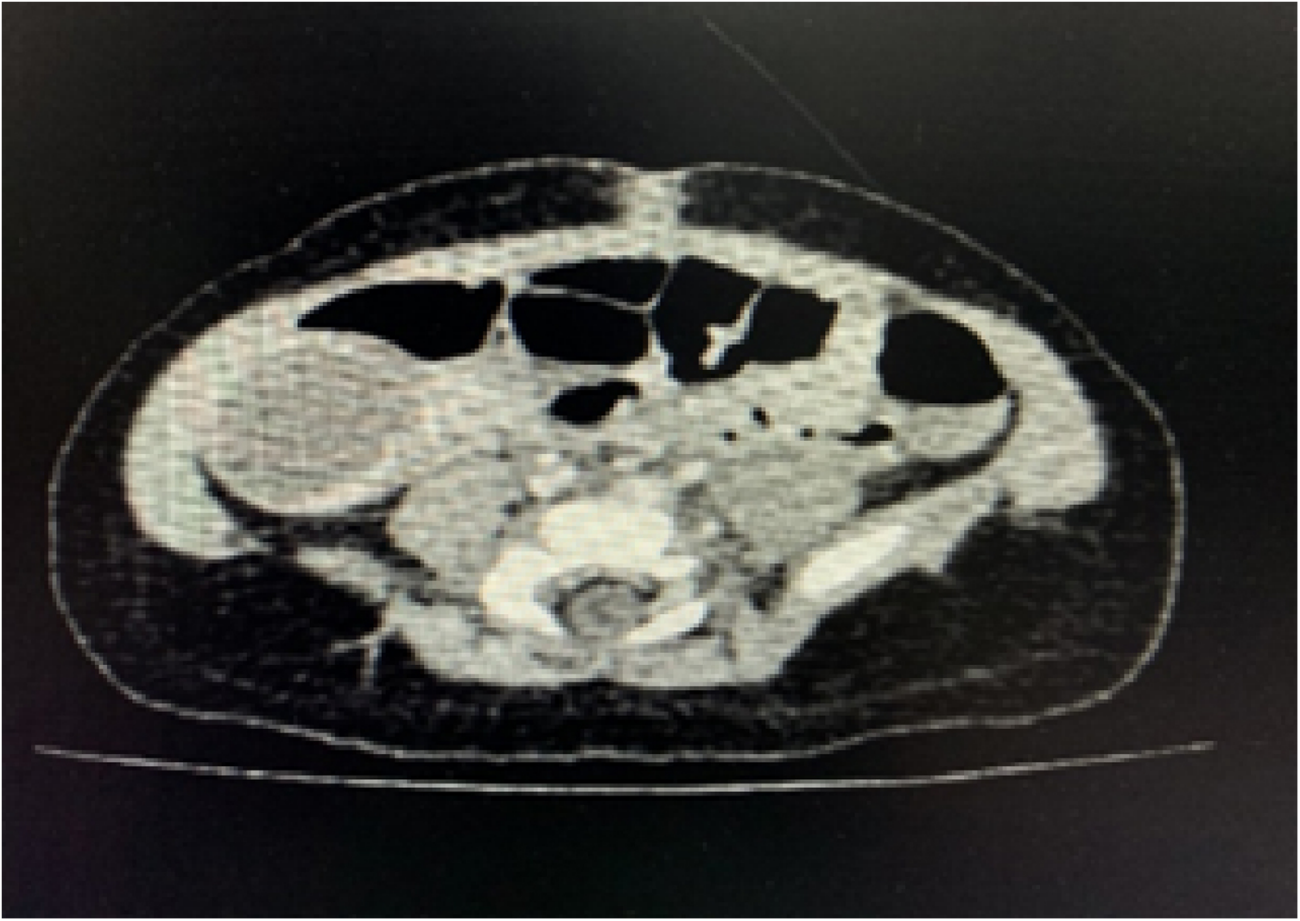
Abdominal enhancement CT: oval-like cystic mass was seen in the right lower quadrant, with a size of 25*30*22 mm. The CT value of the mass was about 30 HU, with a strip of high density shadow at the edge.
